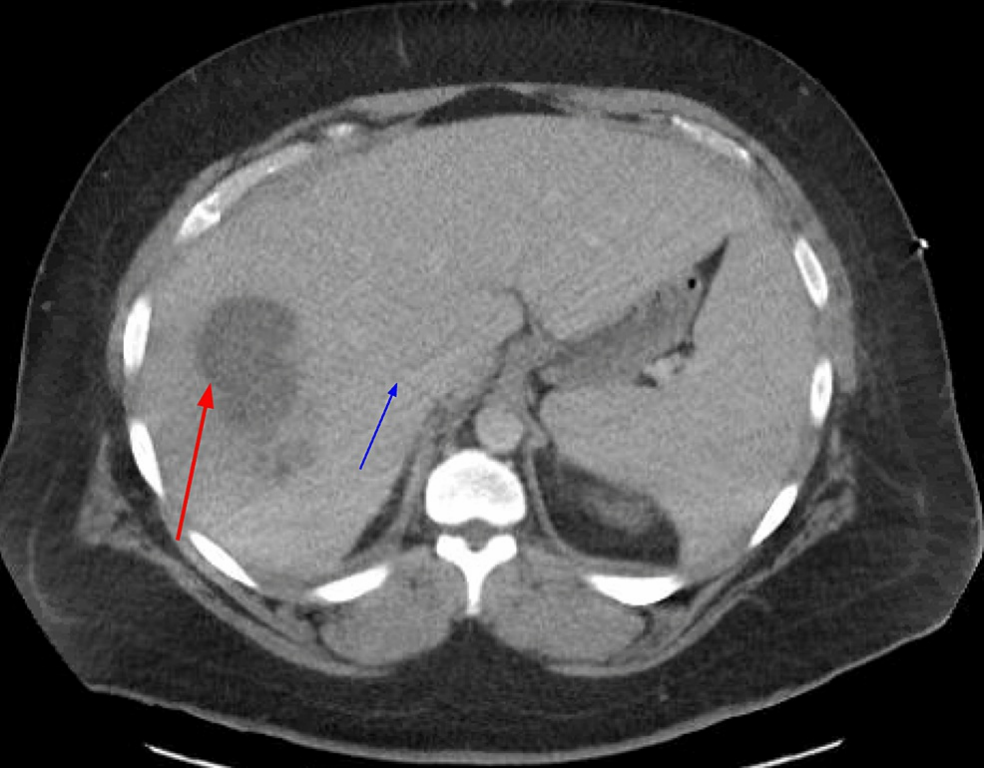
Abdominal computed tomography scan with intravenous contrast image showing hepatic abscess (red arrow) with a linear area of attenuation indicating opacification of a hepatic vein (blue arrow).
